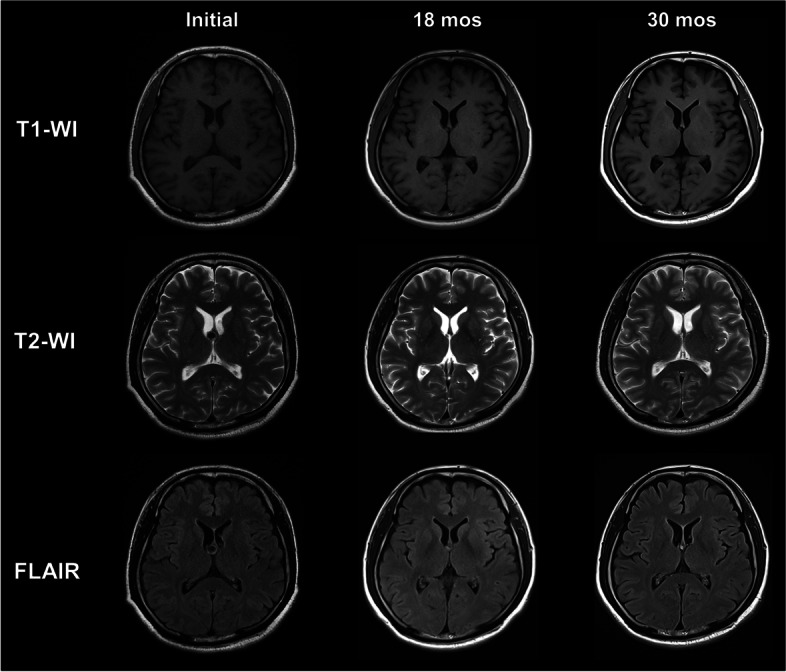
Chronologic evolution of the signal and morphology of a colloid cyst on serial MRI (T1- & T2-weighted, and FLAIR images). Follow-up MRI showed significant regression of the colloid cyst from 12 mm to 4 mm at 18 months after the initial diagnosis. This regression was maintained in follow-up MRI at 30 months
